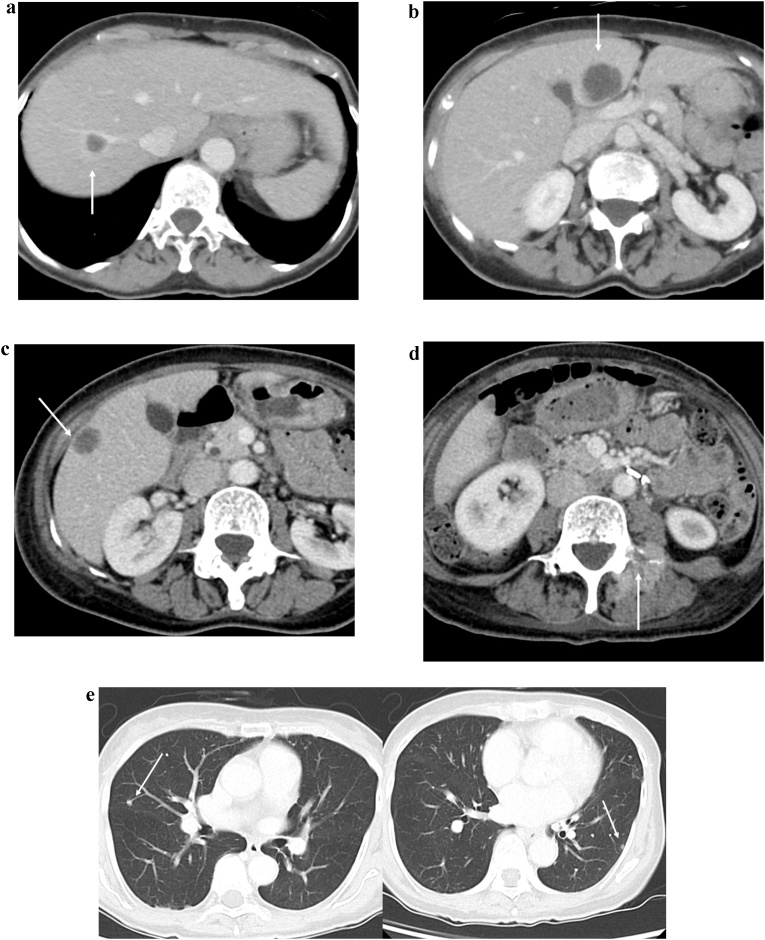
Abdominal contrast-enhanced computed CT findings 3 weeks after surgery. a–c: Few low-density areas with irregular peripheral rim enhancement in the liver. d: 3/4^th^ of lumbar spine melted. e: Multiple pulmonary nodules detected bilaterally (white arrow).
